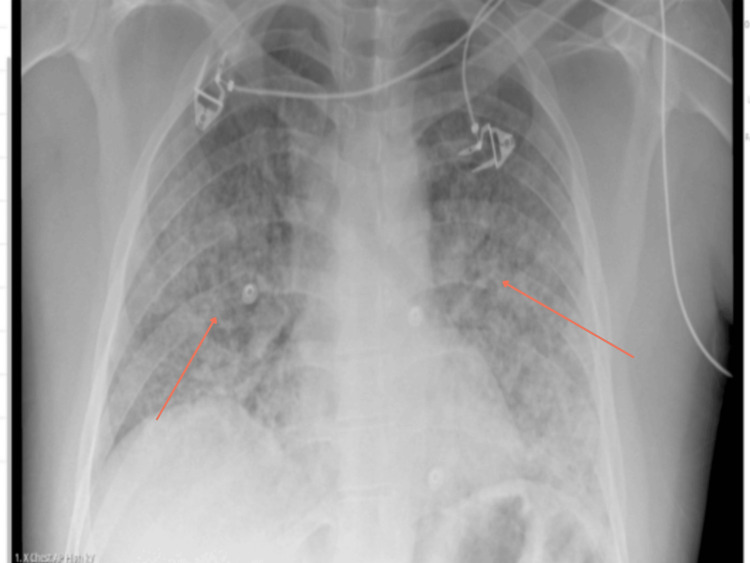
Initial Chest X-ray showing patchy opacification bilaterally suggestive of infection
